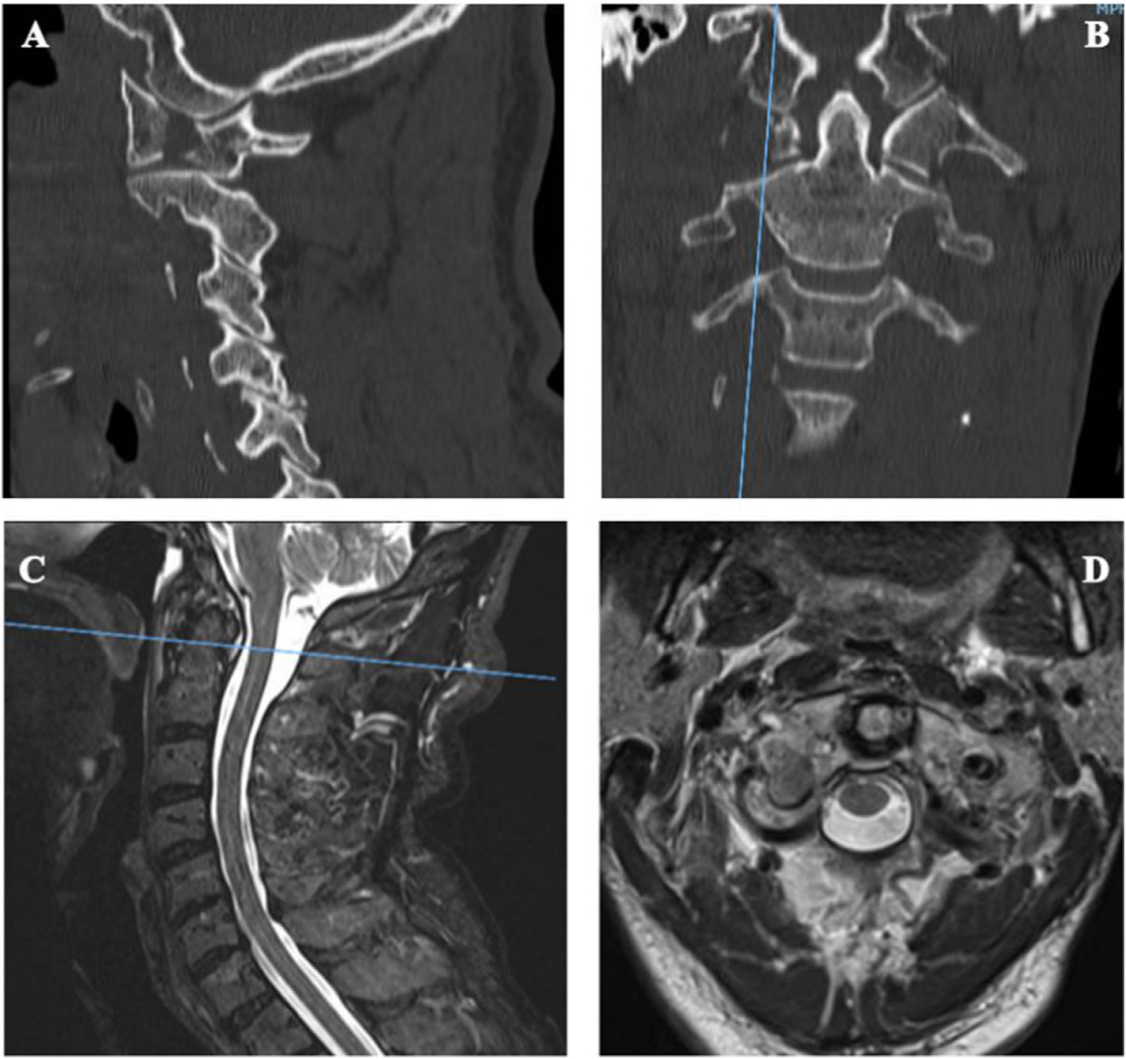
(A–B) CT scan pre-op sagittal and coronal view respectively; (C–D) Cervical-MR: demonstrate no ligament damage.
